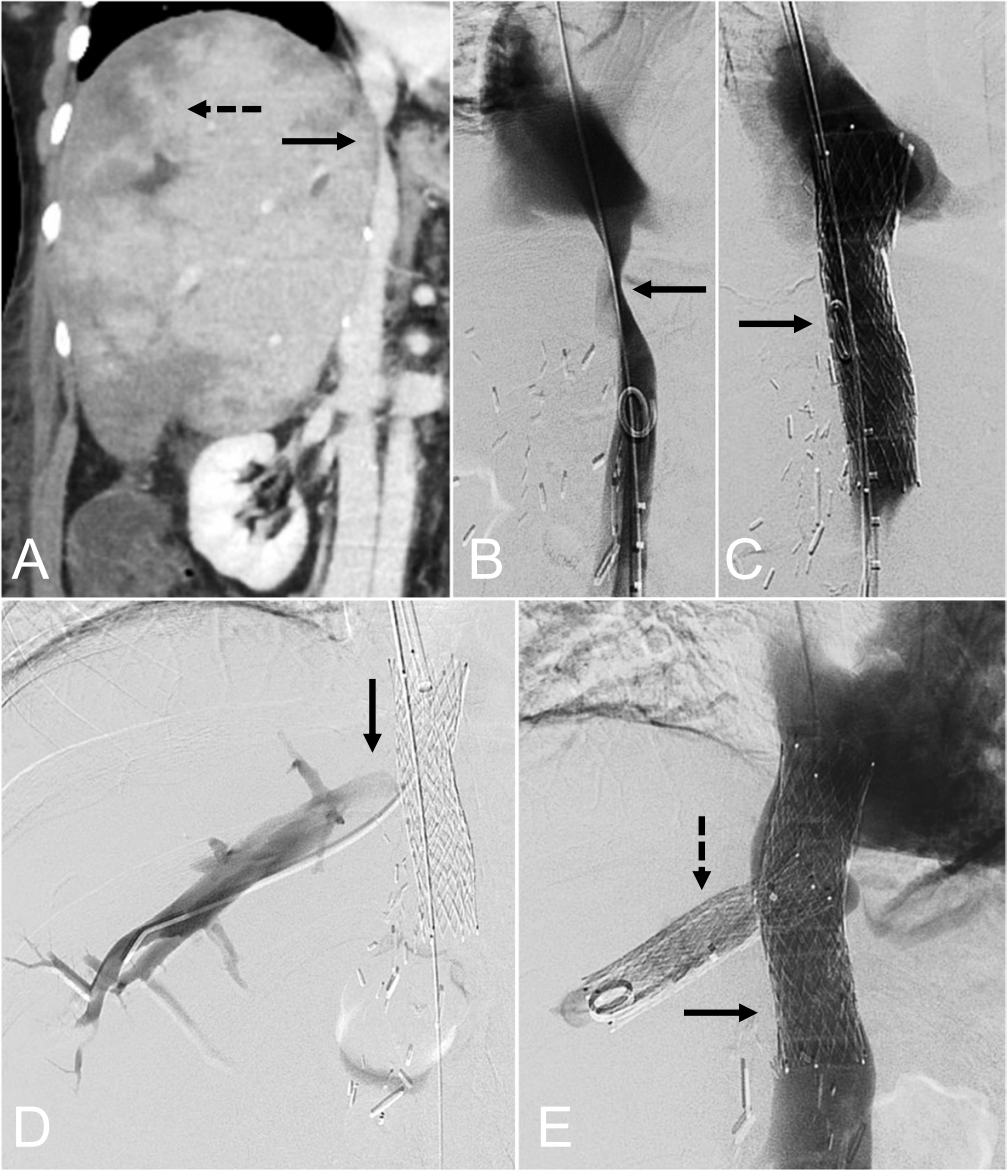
69-year-old male with alcoholic cirrhosis and HCC requiring orthotopic liver transplantation with piggyback anastomosis presented with ascites and transaminitis, and found to have IVC stenosis [solid arrow] resulting in outflow obstruction and hepatic congestion [dotted arrow] on contrast-enhanced CT (A). Diagnostic venography further confirmed IVC stenosis (B), which resolved with stenting utilizing a Wallstent (C). Further selection of the right hepatic vein through the IVC stent was achieved (D), and a right hepatic vein Wallstent was placed through the primary stent (E)
